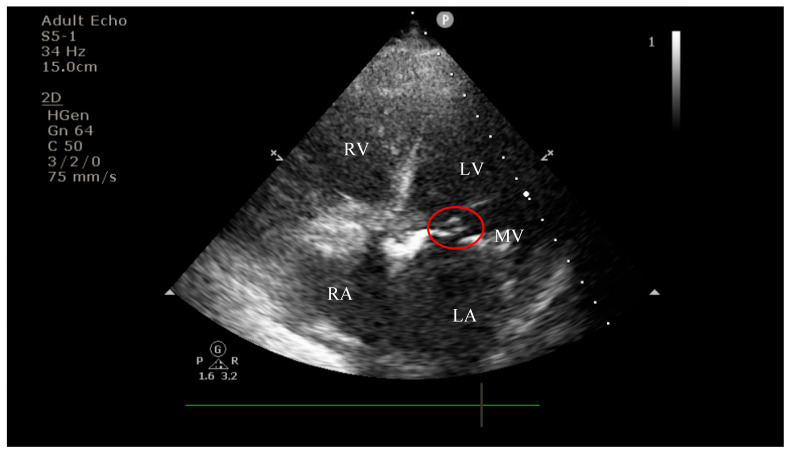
Apical four-chamber view showing a hyperechogenic, mobile, irregular mass attached to the mitral valve; LV—left ventricle; RV—right ventricle; LA—left atrium; RA—right atrium; MV—mitral valve; red circle—hyperechogenic, mobile, irregular mass attached to the mitral valve, measuring 8.5 × 8.2 mm.
